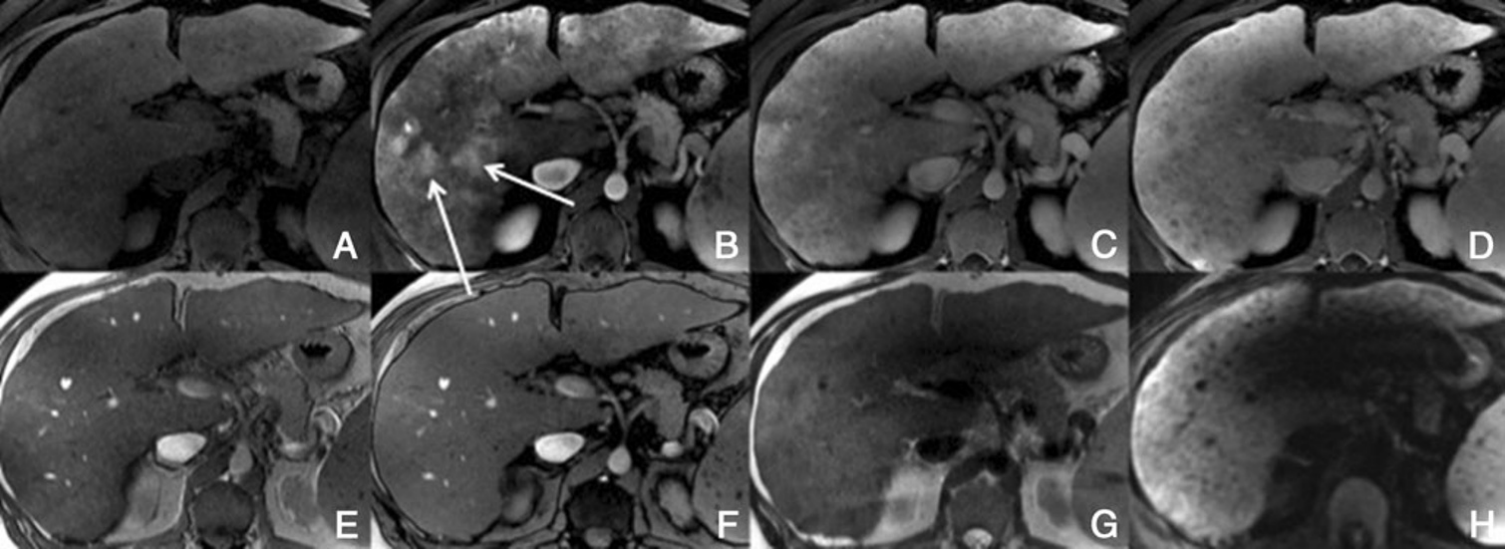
THIDs in a 38-year-old man with chronic liver disease. a Pre-contrast MRI, b arterial phase, c 2 min, d 3 min, e IP, f OP, g T2, h DWI B500. Heterogeneous liver with nodular contour. Fluffy hyperintense areas in the arterial phase (*arrows*), not seen in the other sequences
